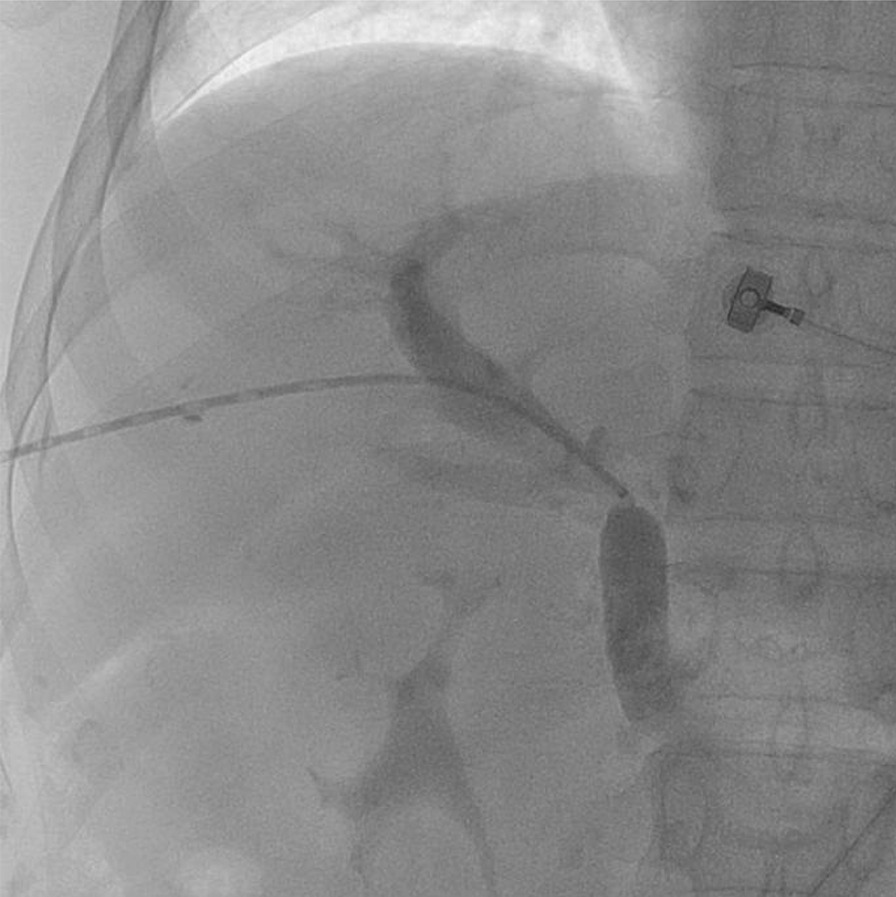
DSA shows severe stenosis of the right and left branches of the portal vein, consistent with the CT showing the site of compression of the lesion
